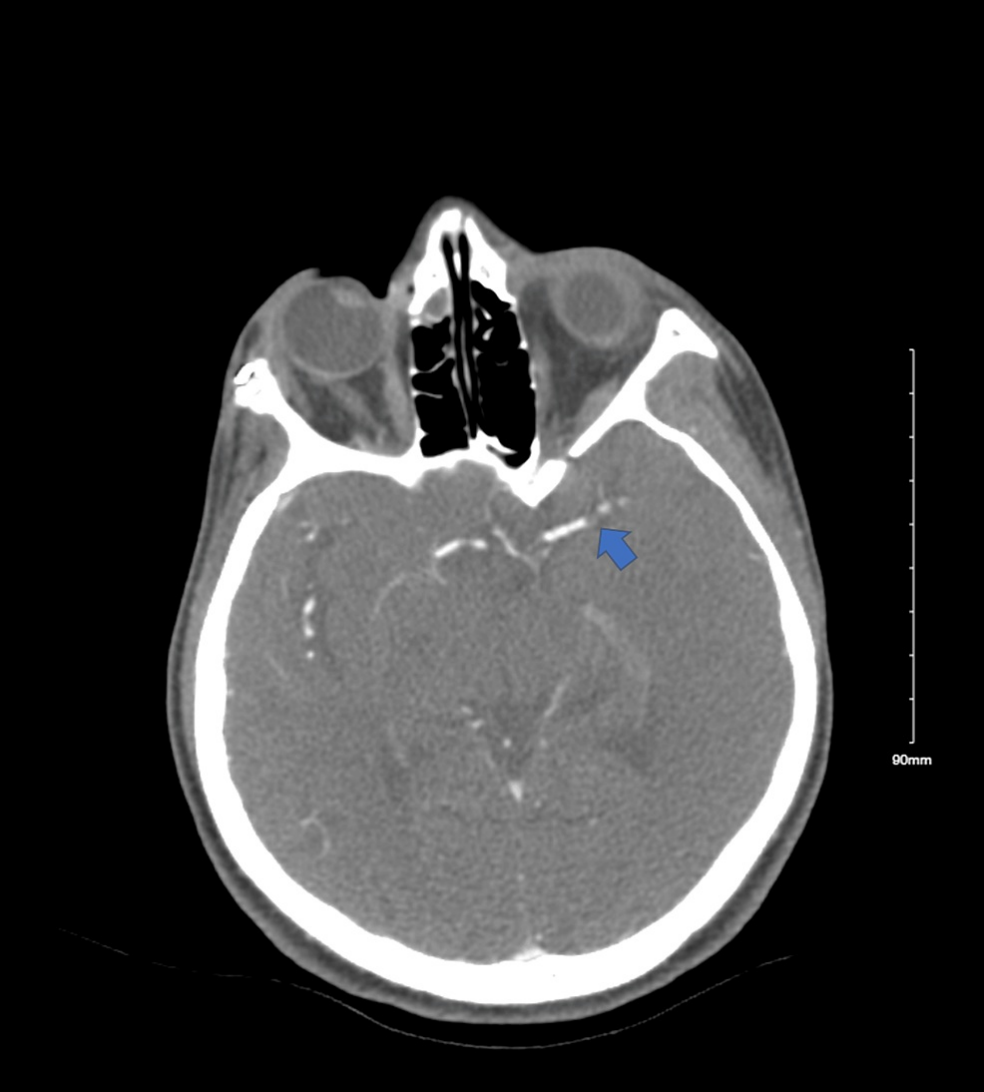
A computed tomography angiogram depicting an occlusion in the M1 region of the middle cerebral artery (blue arrow)
